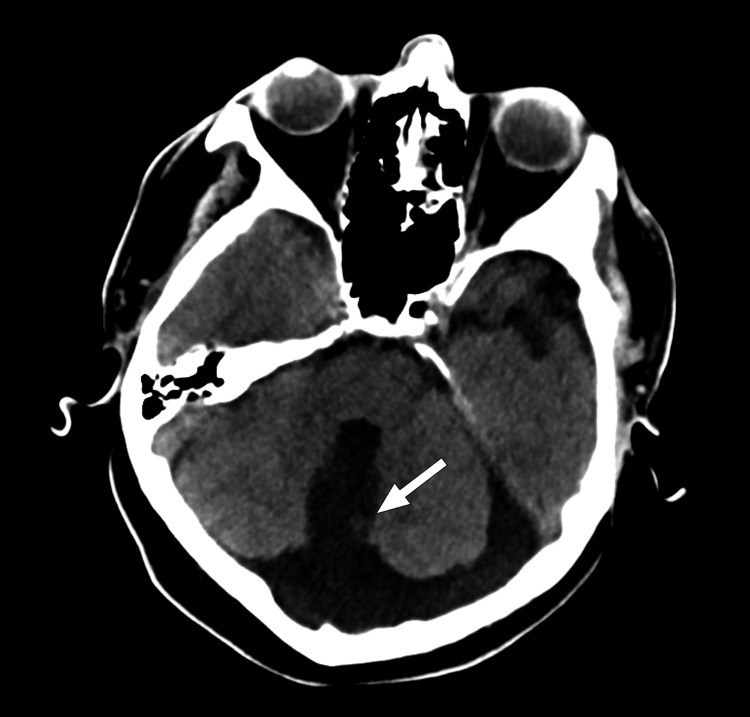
Axial CT of the brain at the level of the fourth ventricle. The Dandy-Walker malformation comprising the fourth ventricle connecting (white arrow) with the posterior fossa cyst is visualized in this view.
